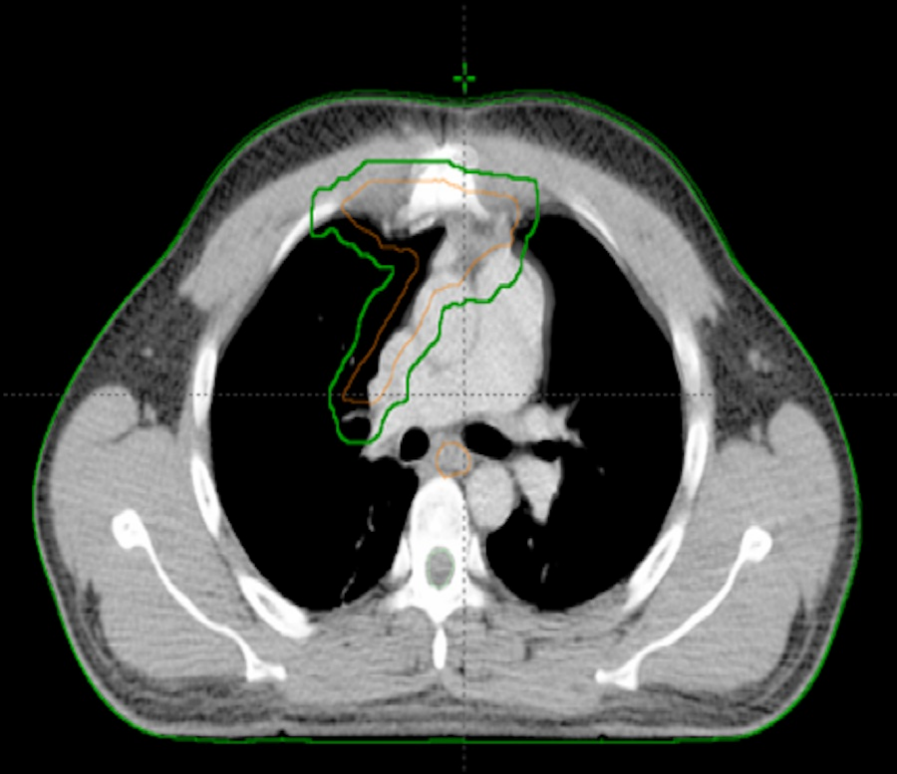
Axial computed tomography image from the radiotherapy planning study with an outline of the clinical target volume (ORANGE) and the planning target volume (GREEN).
